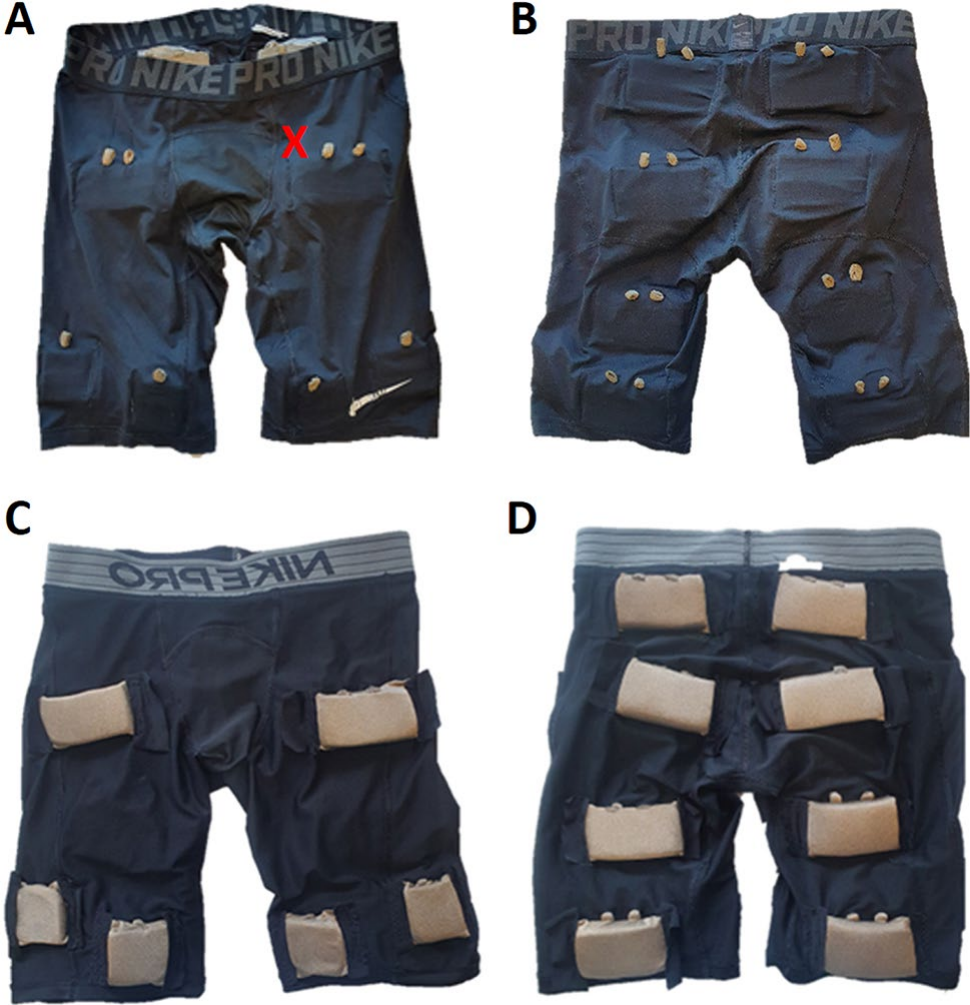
A, B Pants from the outside (A frontside, B backside), seen are the connectors for external junctions. C, D Inside of the pants (C frontside, D backside). Seen are the side of the electrodes that are applied to the skin, the larger electrodes are sized 5 × 9 cm (upper electrodes in C and all electrodes in D) and the smaller (lower electrodes in C, which were used in this study) are sized 5 × 5 cm. The red “X” in picture A demonstrated the approximate location of the ultrasound probe. The pants are thigh fitting and were adjusted so they had good contact with the skin for all participants
